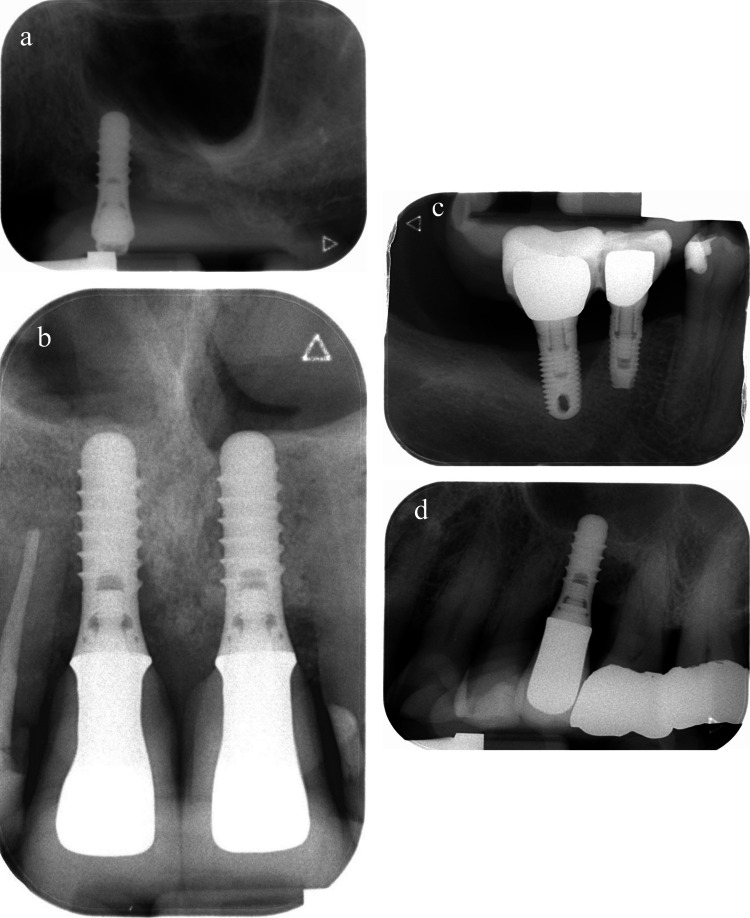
Baseline intra-oral radiographs of the failed test implants. a: Subject 11, b: Subject 23, c: Subject 31, d: Subject 35
